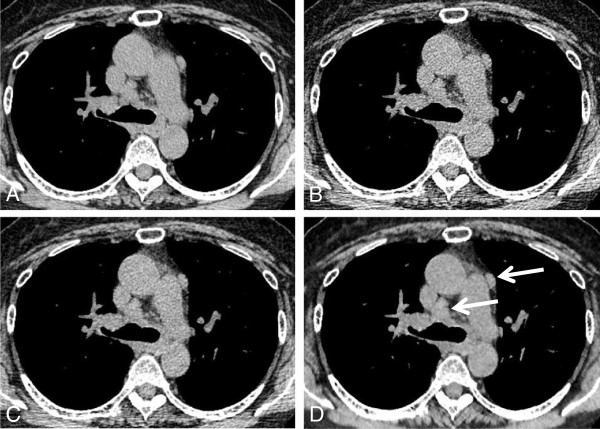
Transverse chest CT through the ascending aorta in a 64 year-old woman with mediastinal lymph node enlargement (arrows). Images were obtained with standard-dose FBP CT (A), low-dose FBP CT (B), low-dose ASIR 50% (C), and low-dose MBIR method (D). Note the excellent depiction of mediastinal lymph nodes on the low-dose MBIR image (lesion conspicuity score 5), compared with low-dose CT with FBP (score 3) and ASIR (score 4). Objective image noise on low-dose MBIR CT is 12.12 HU, showing higher than those on standard-dose FBP (17.72 HU), low-dose FBP (27.69 HU), and low-dose ASIR CT (20.76 HU) in this patient.
